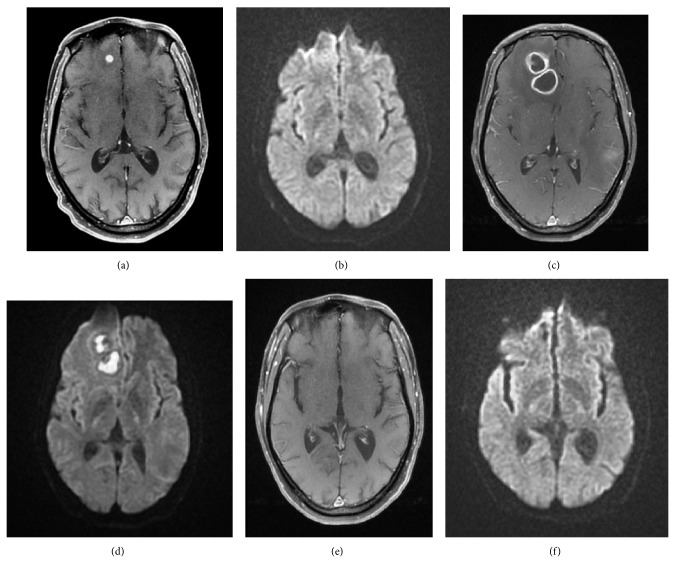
Single small nodular enhancing lesion with discrete central hyposignal (a) not seen on DWI (b). Two ring enhancing lesions (c), with partial restricted diffusion (d). No lesions seen on T1 WI post gad (e) and DWI (f).
